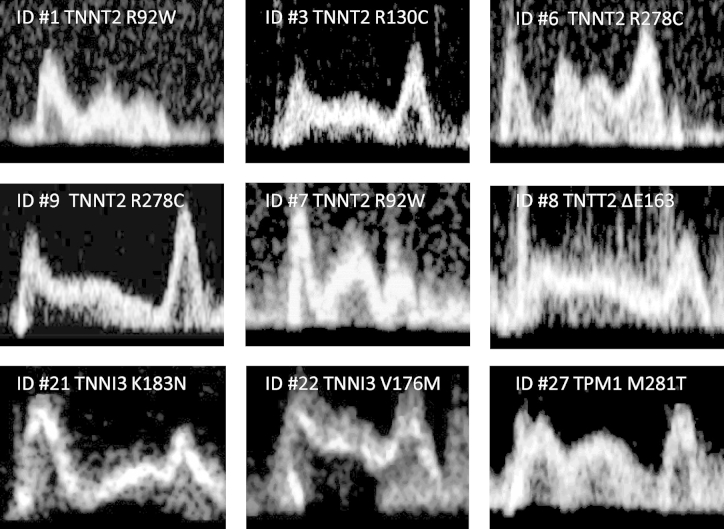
Triphasic LV Filling Pattern Representative transmitral blood flow velocity patterns assessed by pulsed-wave Doppler echocardiography in 9 patients with thin-filament–associated hypertrophic cardiomyopathy (patient ID numbers and mutations are shown). Mid-diastolic flow velocity (L-wave) is clearly visible (*). An L wave’s presence is independent of the overall diastolic pattern, with E/A ratios that can be <1 (i.e., delayed relaxation) or >1 (i.e., pseudonormalized). LV = left ventricular.
